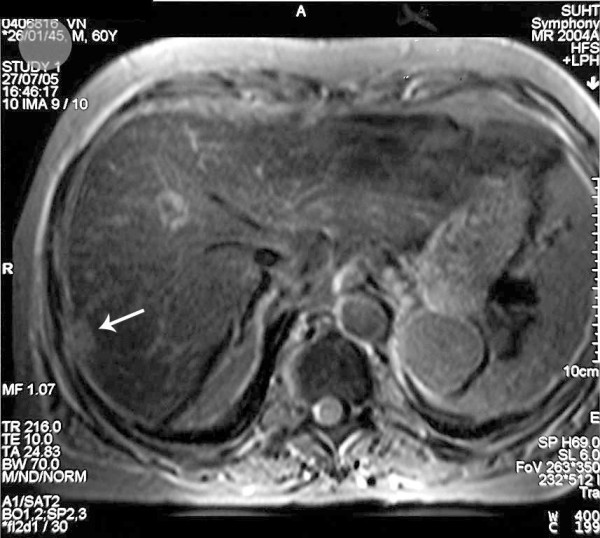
Post Resovet Axial MRI images. The segment VII lesion demonstrates higher signal than the background, reflecting a relative lack of functioning hepatocytes.
